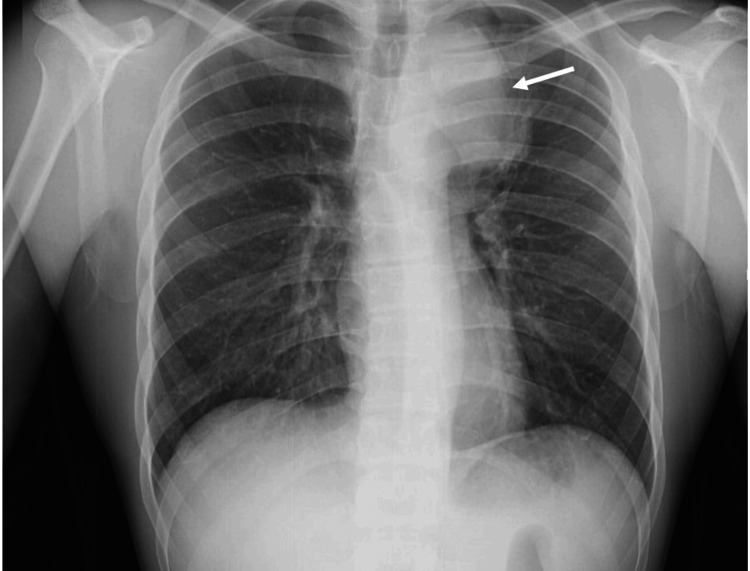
Left paratracheal opacity on chest roentgenogram (white arrow)
